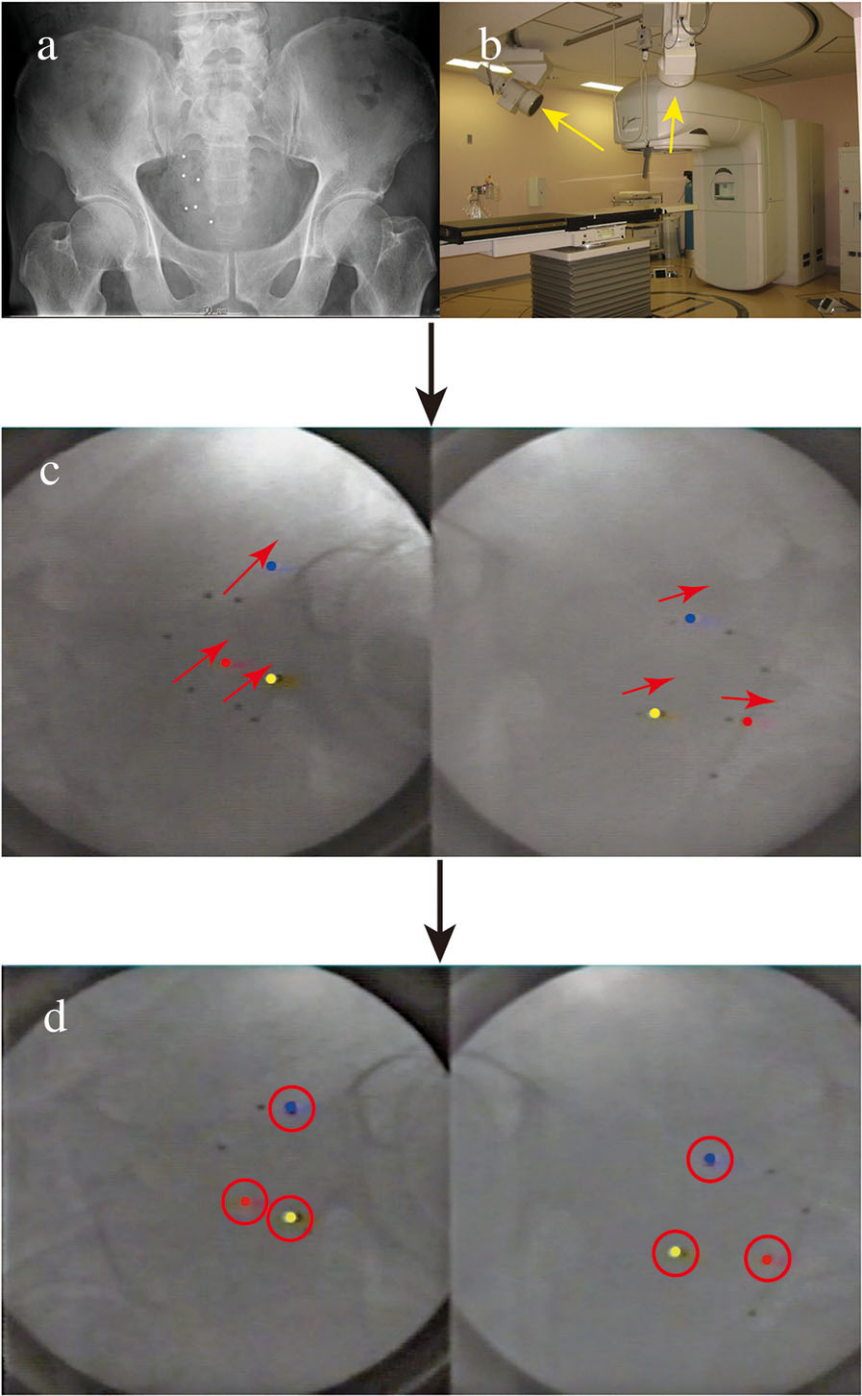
Example of fiducial marker registration. a X-ray of patient abdomen with the tumor located in the right bladder wall. This patient had 6 gold markers implanted around the transurethral resection of the bladder tumor (TUR-BT) scar. b Arrangement of the fluoroscopic units with the direction of the X-ray beam (arrows). The images were acquired by the two units that are a part of the RTRT system. c The colored circles in the fluoroscopic image indicate the gold marker positions at the registration and the black dots represent the actual positions of the gold markers. Up to three markers could be displayed in this system. The red arrow indicates the direction of the required positional correction. d Fluoroscopic images after fiducial marker registration. The red circles indicate the fiducial markers placed at the planned position
